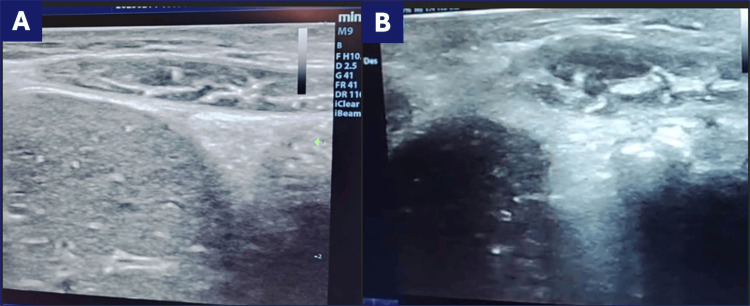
Ultrasound-guided botulinum toxin type A infiltration. (A) Identification of the selected abdominal wall muscle plane under ultrasound guidance. (B) Intramuscular injection of botulinum toxin type A, with local separation of the muscle fibers confirming delivery within the intended plane.
